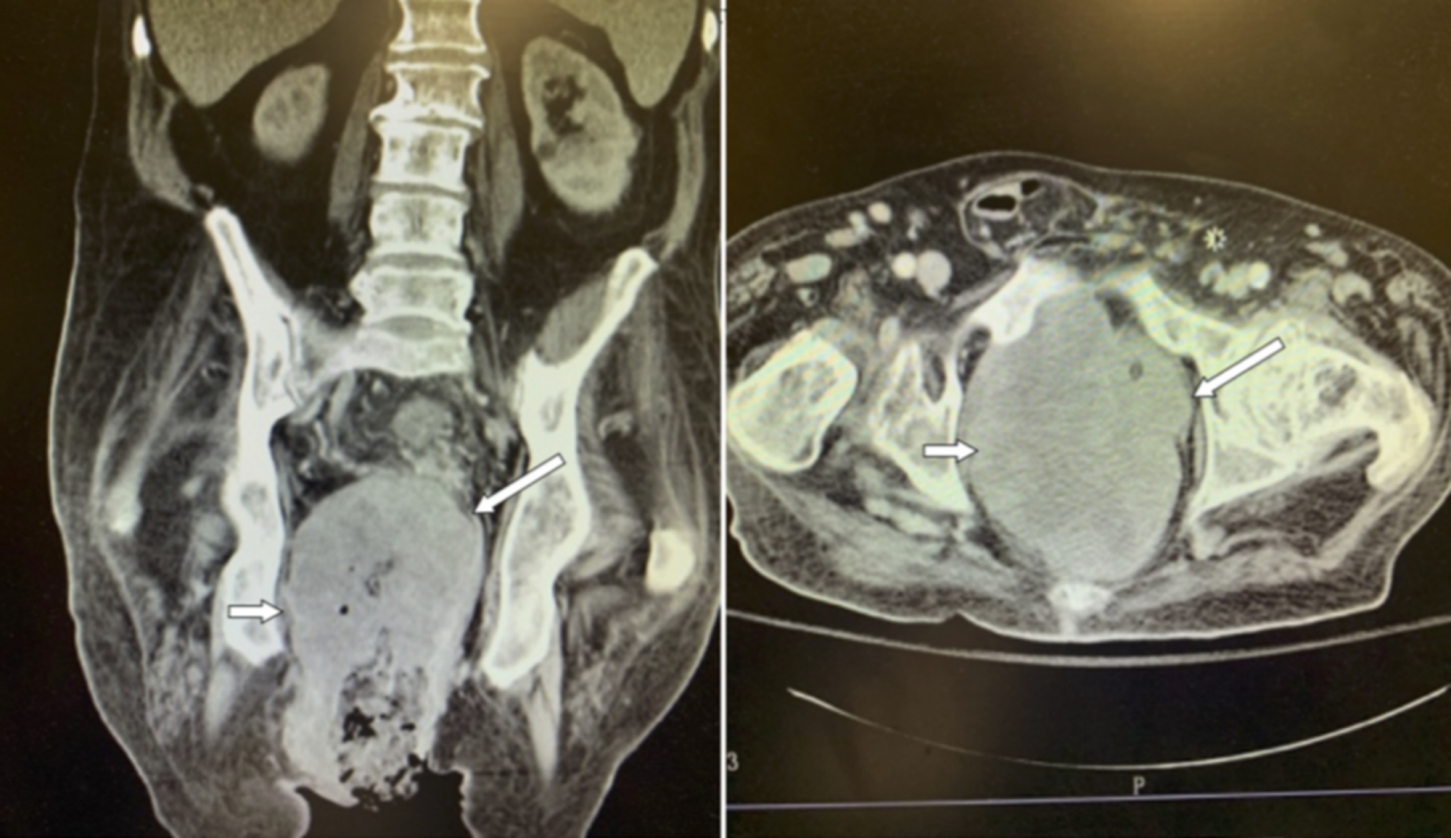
Computed tomography on readmission, showing the complex abscess cavity (long arrow) and mass lesion (short arrow)
